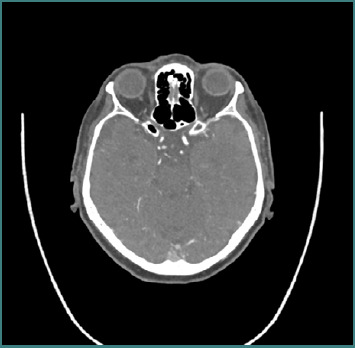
CT angiography demonstrated basilar artery occlusion (BAO)
